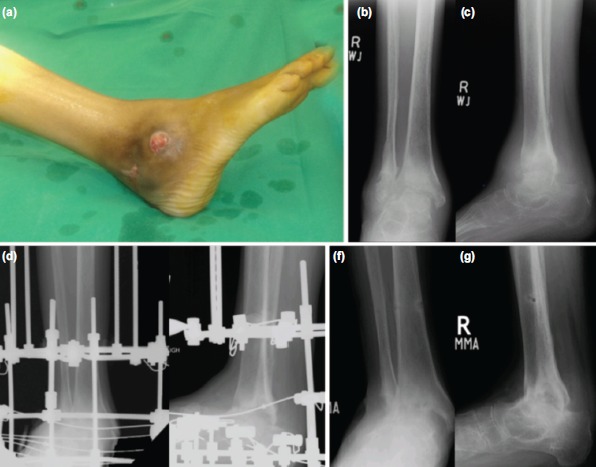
(a) Intra-operative photograph of 45 year-old lady with tuberculosis ankle joint showing ulcers at ankle. (b,c) Pre-operative radiographs Ilizarov anteroposterior and lateral views showing subchondral erosions and sclerosis. (d,e) Immediate postoperative radiographs showing Ilizarov fixator across ankle joint Ilizarov anteroposterior and lateral views. (f,g) Nine months post-operative radiographs Ilizarov anteroposterior and lateral views after removal of Ilizarov fixator showing ankle joint fusion.
